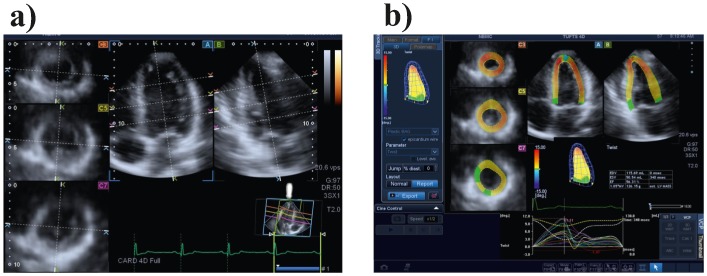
3D Speckle Tracking Ecocardiography (3D-STE). a) manual identification of homologous planes and landmarks on the echocardiographic image; b) subsequent automatic generation of a series of semi-automated landmarks on the endo- and epicardium; color map shows the amount of Twist in different cross sections of the LV as computed by postprocessing the position data of all landmarks.
